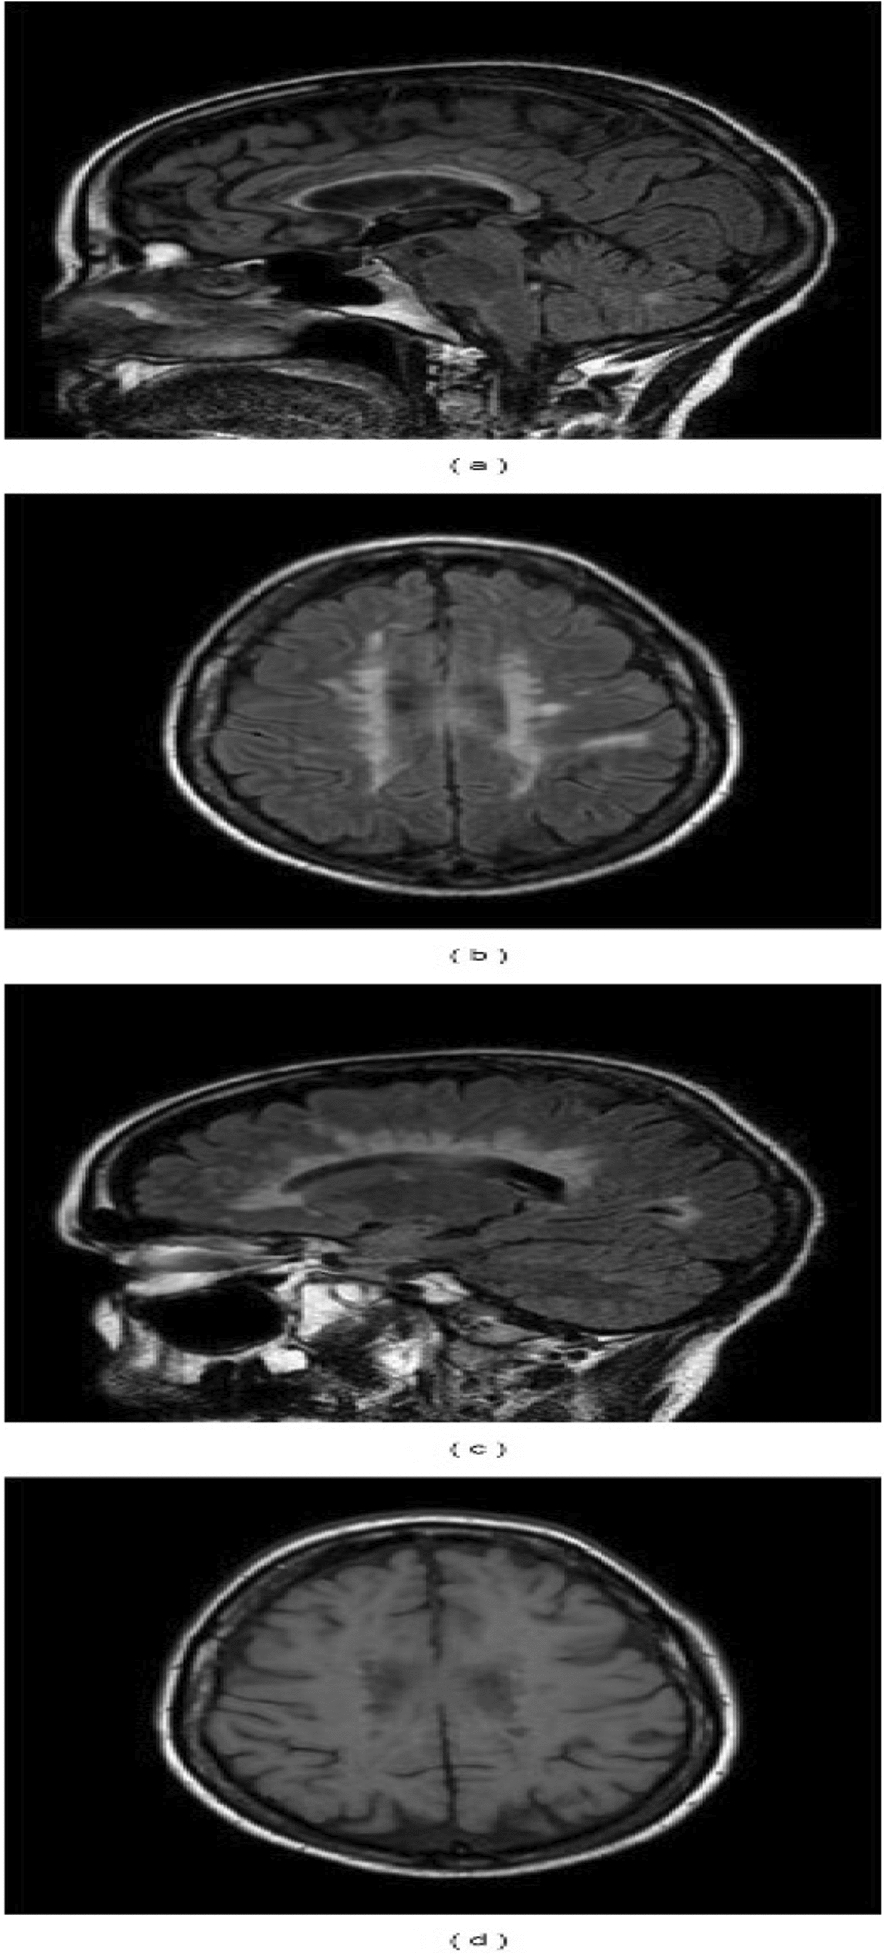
Sagittal (a), axial (b), and parasagittal (c) FLAIR MRI scans showing corpus callosum involvement. T1 axial scan (d) shows the presence of numerous T1 hypointense lesions (“black holes”). [34] Copyright © 2014 A. Lunardelli et al.
